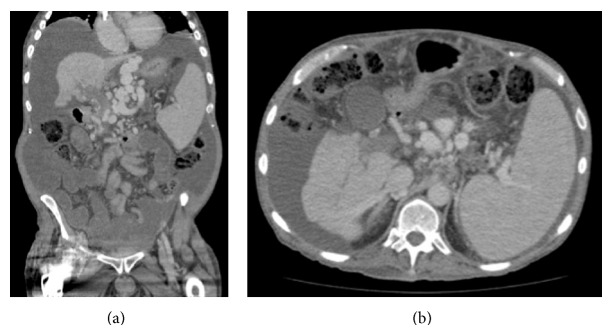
(a) CT abdomen/pelvis with and without contrast in coronal portal phase showing complete occlusion of the entire portal vein at the level of the confluence of the superior mesenteric vein and mild cavernous transformation of the main portal vein. Splenomegaly noted, measuring 19.6 cm in craniocaudal dimension with moderate to large ascites. (b) CT abdomen/pelvis with and without contrast in axial portal phase showing a cirrhotic liver with no arterial hyperenhancing hepatic lesion or lesion with washout. There are multiple calcifications in the main pancreatic duct at the neck resulting in dilatation of the pancreatic duct involving the body and tail measuring up to 1.3 cm.
